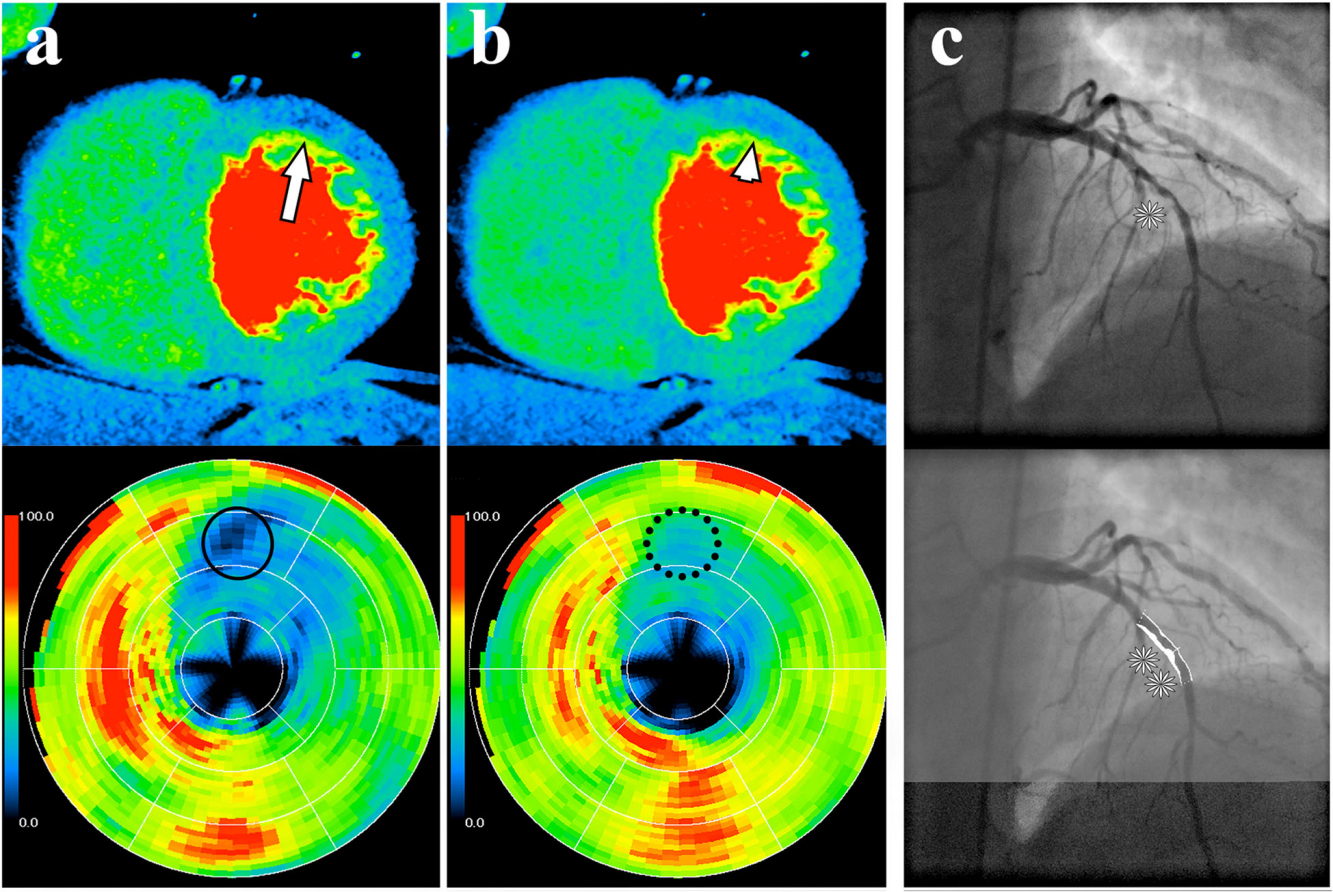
HSR and two-segment MSR of stress myocardial CTP in a 55-year-old man with suspected coronary artery disease and typical angina pectoris in comparison with invasive coronary angiography. a HSR shows a moderate perfusion defect in the left anterior descending artery territory (arrow), which is also suggested by moderate hypoattenuation in the corresponding area (circle) of the polar myocardial attenuation map; CTP with HSR was considered positive. b MSR shows only very slightly reduced perfusion (arrowhead) and only weak hypoattenuation in the corresponding area (dotted circle) of the polar myocardial attenuation map; CTP with MSR was considered negative. c Invasive coronary angiography reveals visually high-grade diameter stenosis (*) of the left anterior descending artery with 61% stenosis in quantitative invasive coronary angiography (), corresponding to a true-positive CTP with HSR (a) and a false-negative CTP with MSR (b). Contrast-enhanced CTP in mid-heart short-axis view with 8-mm slice thickness and rainbow-red colour preset using a predefined window level/window width of 200/400. *CTP* Computed tomography perfusion, *HSR* Halfscan reconstruction, *MSR* Multisegment reconstruction
